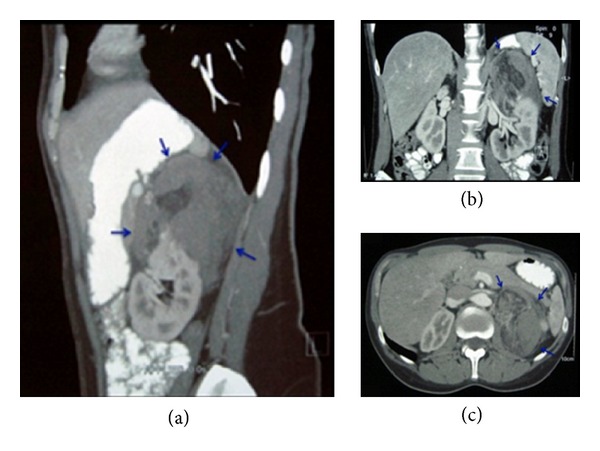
CT imaging of the abdomen depicting AML of the left kidney. (a) Sagittal plane. (b) Coronal plane. (c) Transverse plane.
